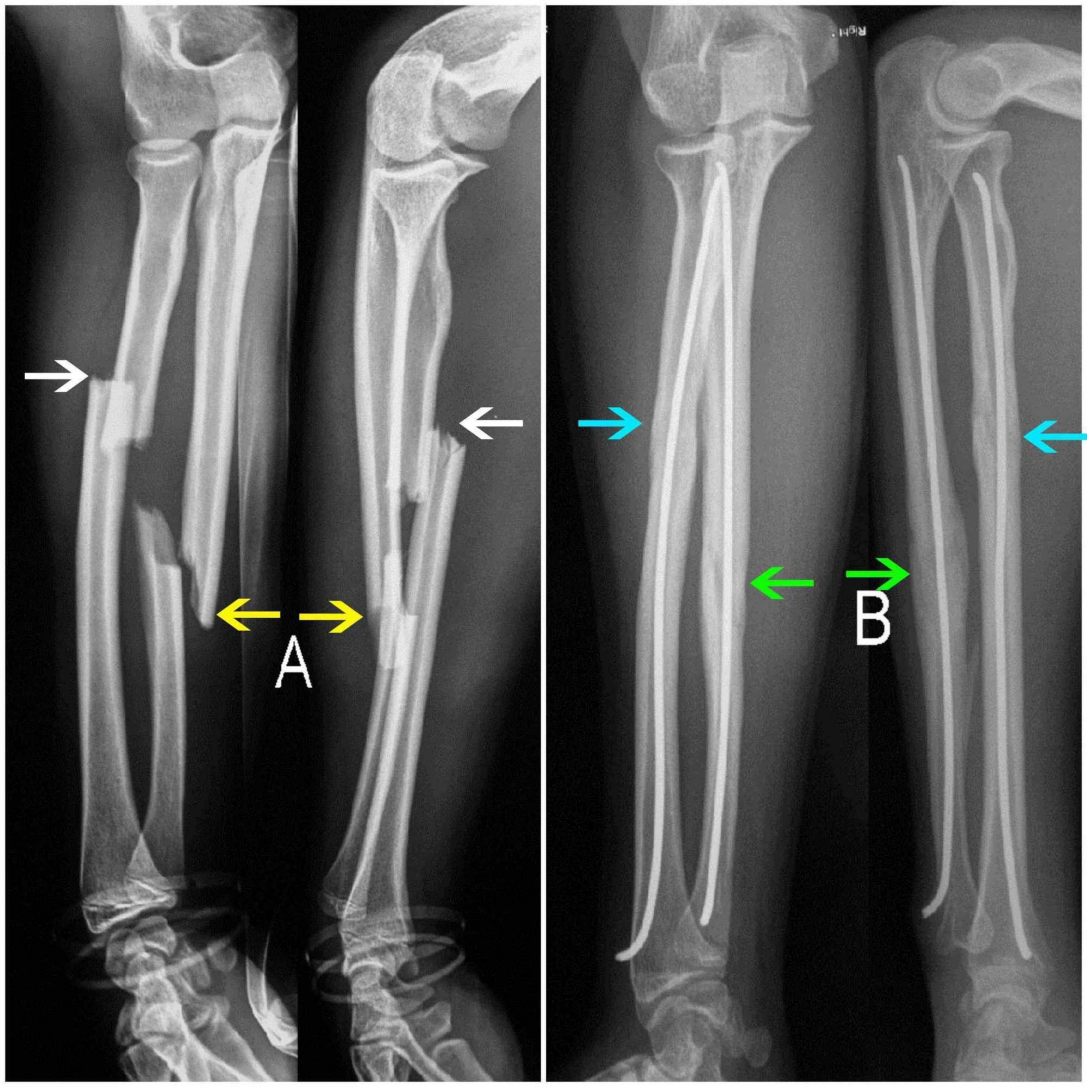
Initial fracture and four-month follow-up (A) Initial X-ray showing fracture of both the radius (white arrows) and the ulna (yellow arrows) in the anteroposterior (AP) and the lateral (L) view. (B) Four-month follow-up X-ray showing healing of both the radius (blue arrows) as well as the ulna (green arrows) in the AP and the L view.
